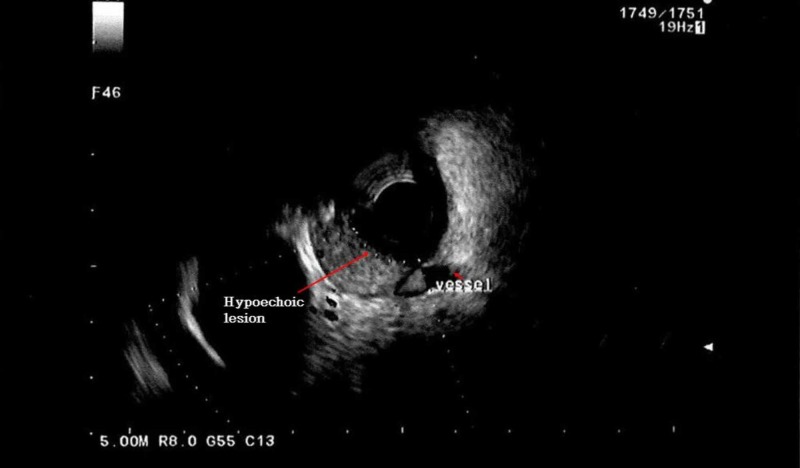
Endoscopic ultrasound shows a hypoechoic lesion arising from the mucosal layer, with a large blood vessel feeding the lesion.
